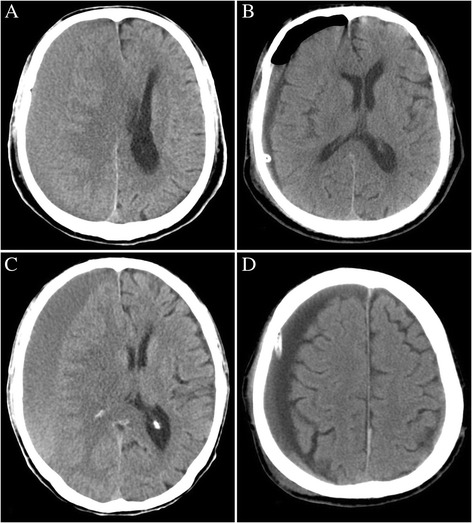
CT scans of two typical patients undergoing BHD and BHDI. a and b are the preoperative and first postoperative day images of a patient receiving BHDI, respectively. c and d are the preoperative and first postoperative day images of a patient receiving BHD, respectively. Obvious pneumocrania can be observed in image b. This image is from the preliminary report of the present study, which was published in West China Medicine Journal [27]. The authors have got the permission to republish this image
